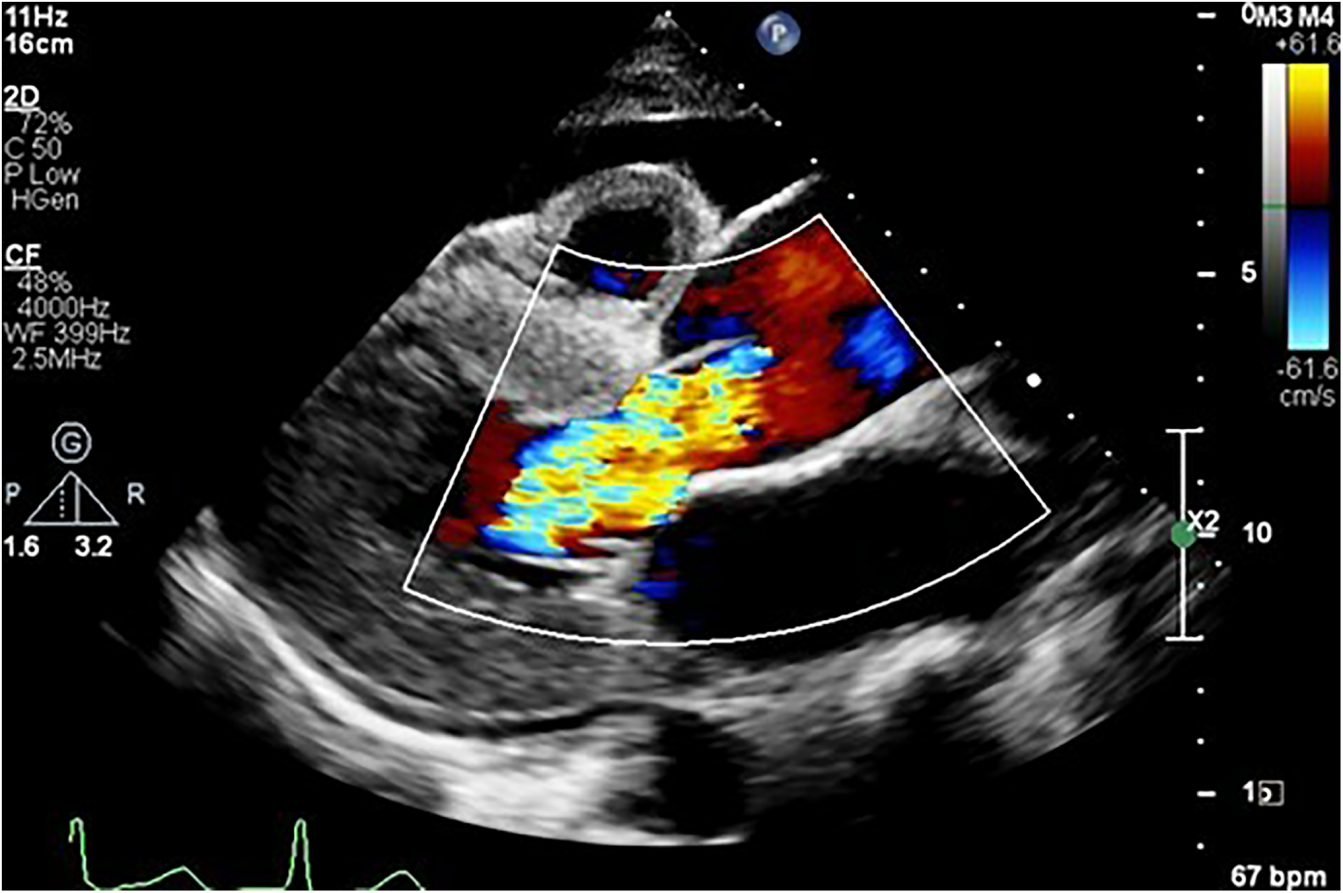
Parasternal long axis view showing an aortic aneurysm secondary to hypertension complicated by heart failure, as evidenced by the anterior pericardial effusion secondary to severe aortic regurgitation.
